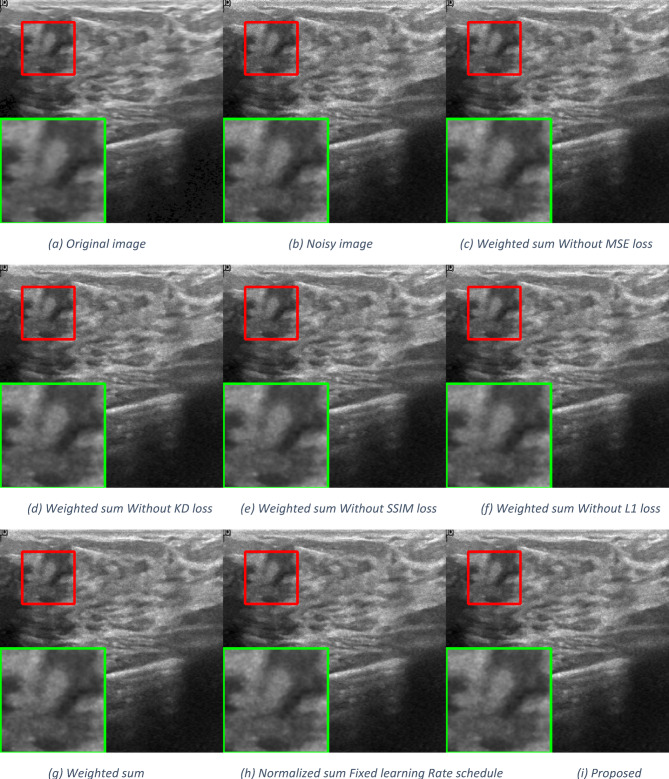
Impact of PSNR-Normalized Loss, Standard Loss, and Learning Rate Reduction on the Breast Ultrasound Dataset (σ = 0.01).
